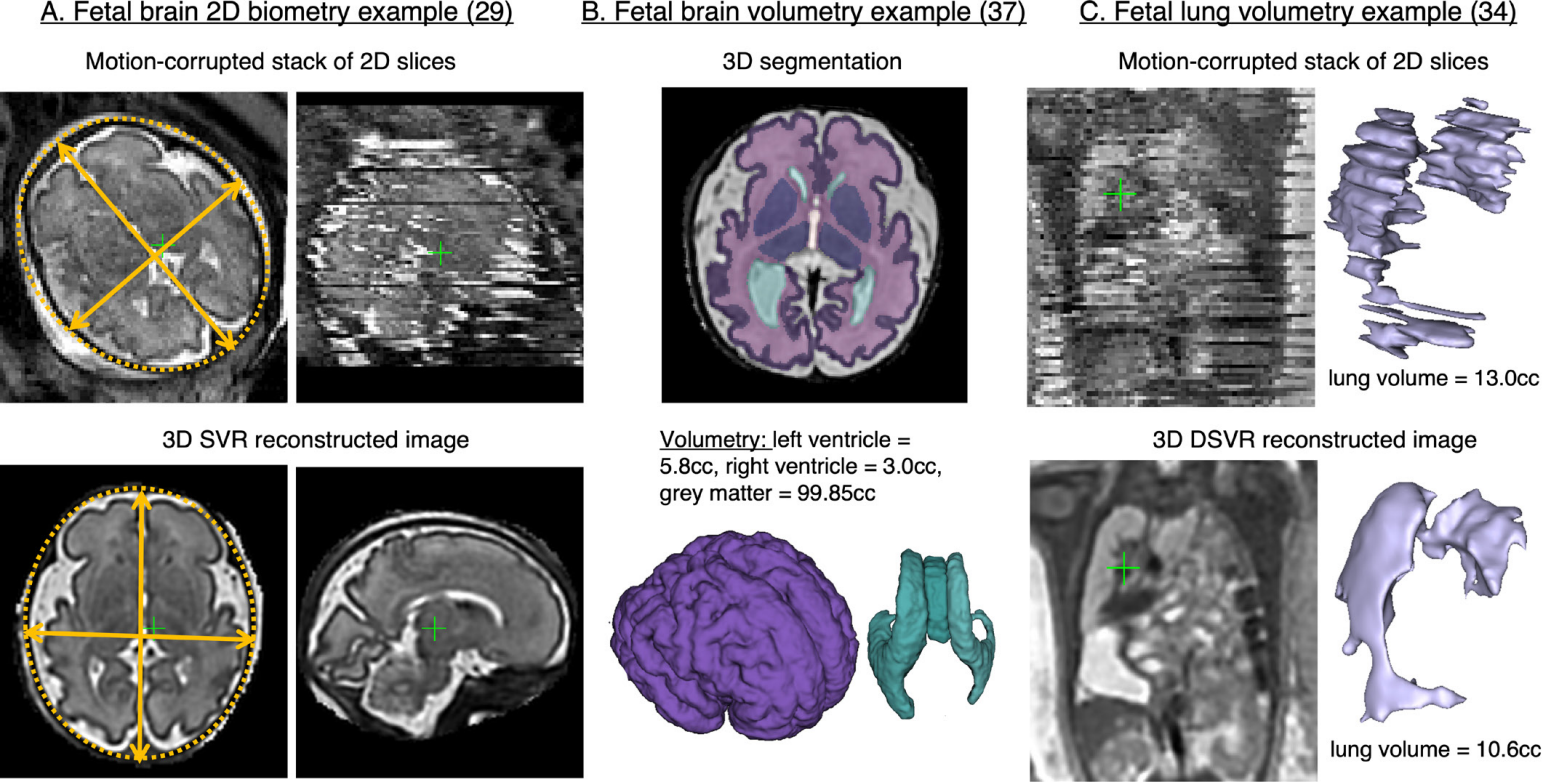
Examples of existing quantitative applications for 3D MRI reconstructed images. The examples are based on the MRI datasets acquired at St.Thomas’ Hospital, London. SVR, slice-to-volume registration.
